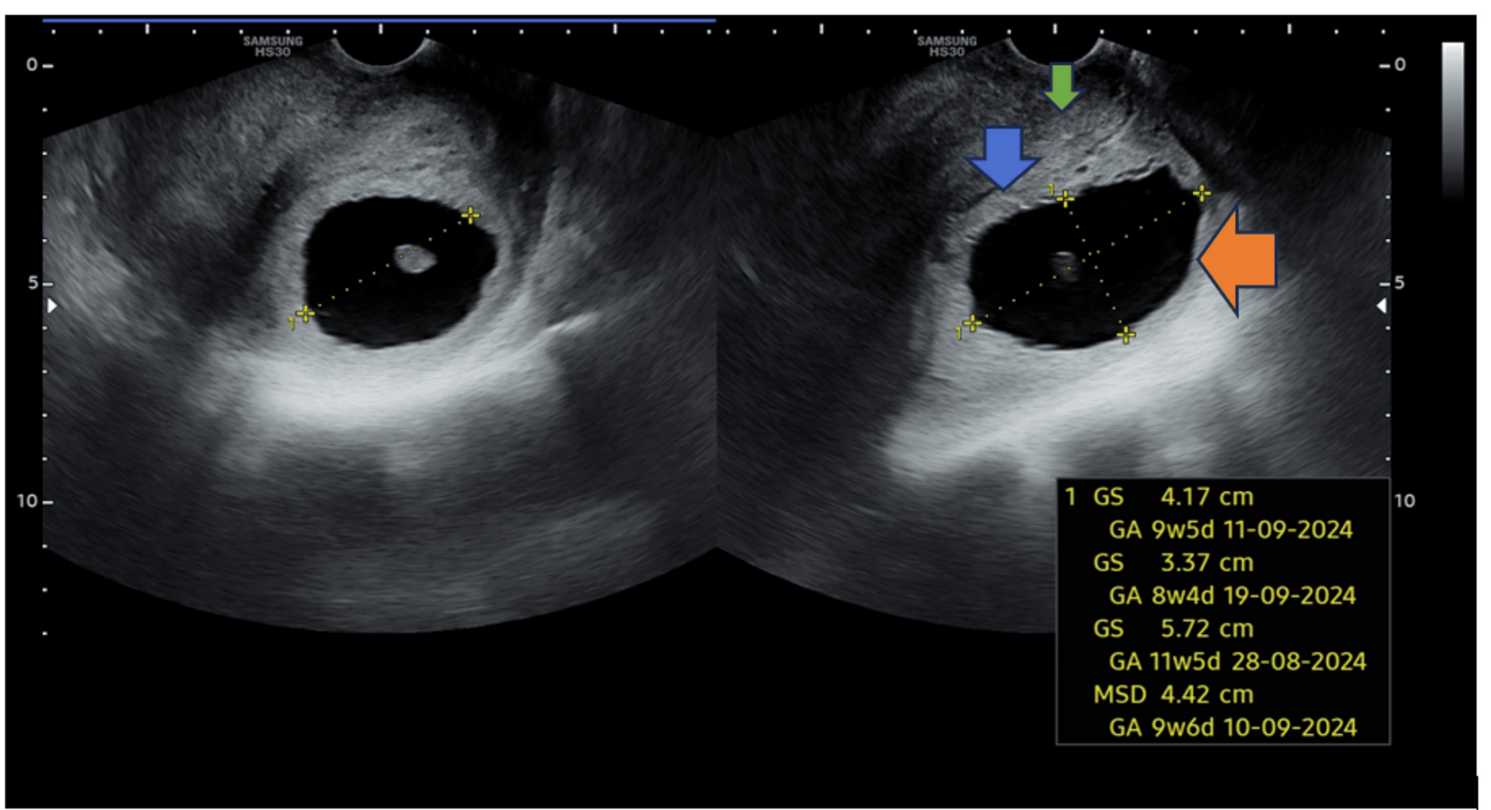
Ultrasound image showing a hypoechoic lesion representing the gestational sac (GS) with a fetal pole. The blue arrow indicates the hyperechoic line of the cervical canal; the orange arrow shows the posterior lip of the cervix with the GS; and the green arrow shows the anterior lip. GS, gestational sac; MSD, mean sac diameter; GA, gestational age
